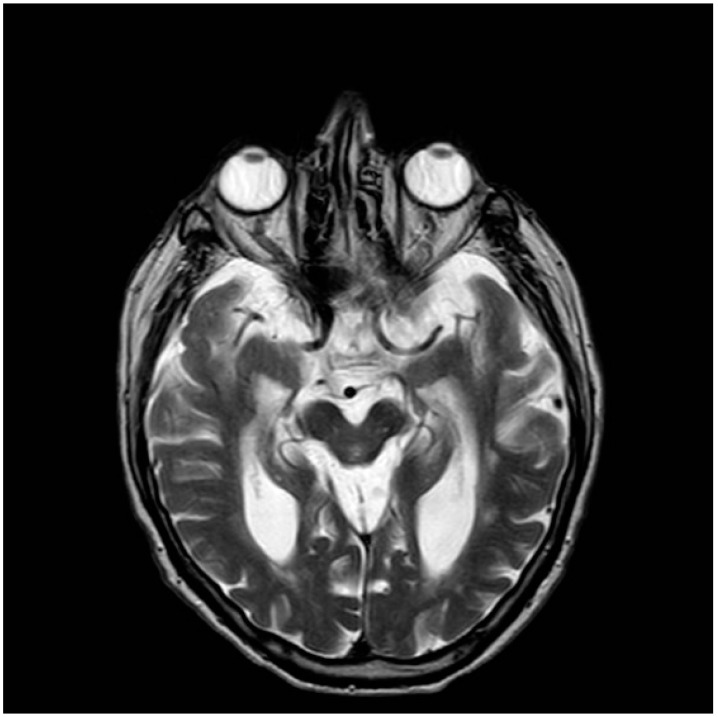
Axial MRI T2 scan demonstrating characteristic morning glory sign in PSP.
